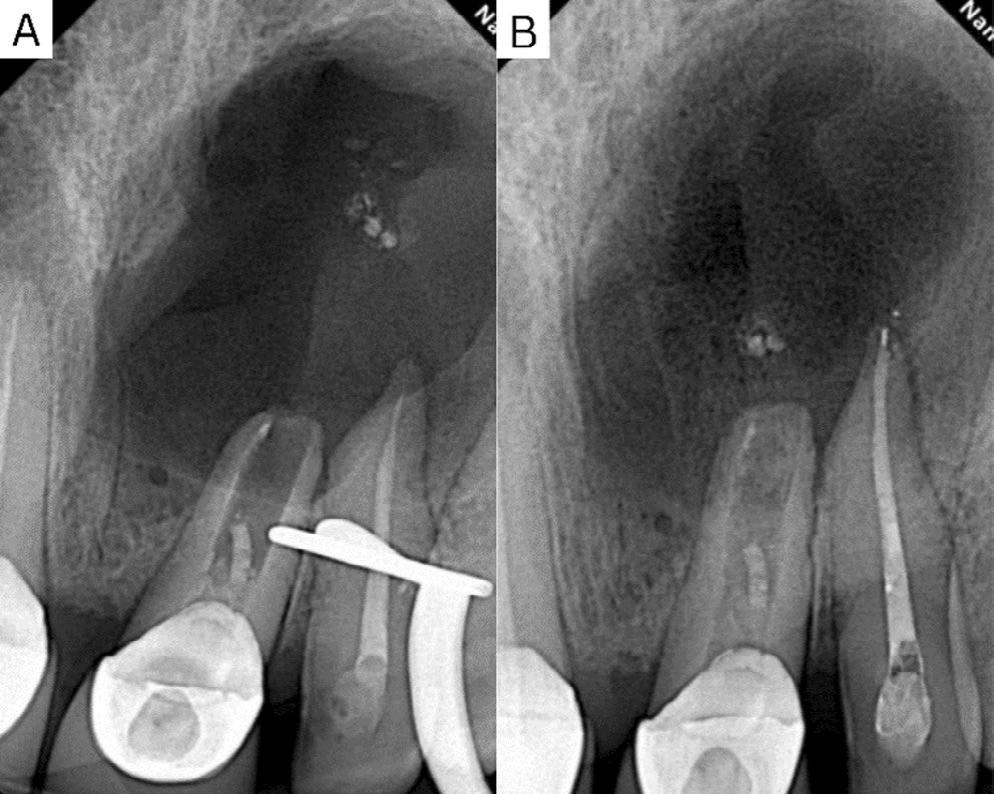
(A) Immediate periapical radiograph after decompression. (B) Follow‐up radiograph after 3 months demonstrating bone healing following pressure relief from the internal lesion. The upper left lateral was also retreated in the meantime due to inadequate past obturation.
